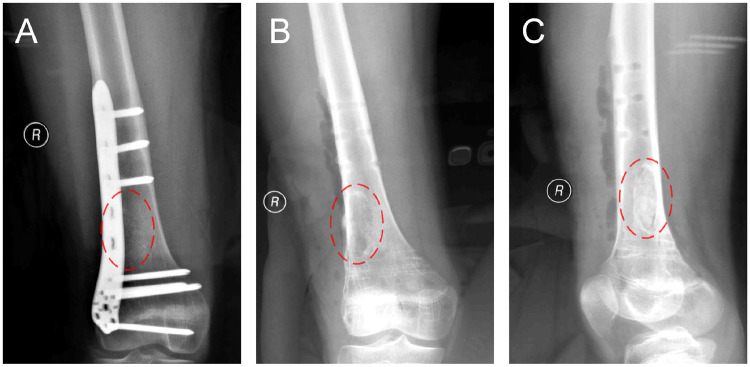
Plain radiography before (A) and after (B, frontal view; C, lateral view) removal of the internal fixation at 9 months after the operation (the surgical site is noted by dashed circle in each picture).
